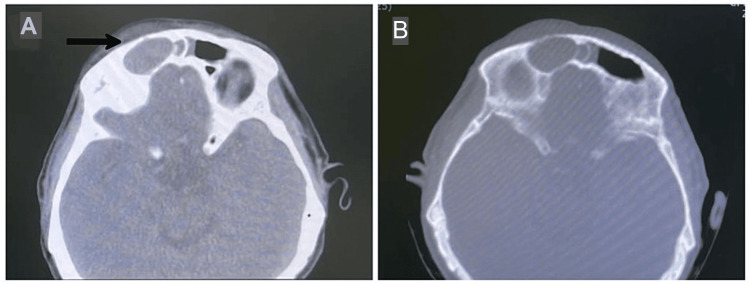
Case 3. Axial CT scan bone window at level of fronto-ethmoidal area showing expansile lesion occupying the right ethmoid (arrow), and right frontal area with intact anterior and posterior table of frontal bone.
